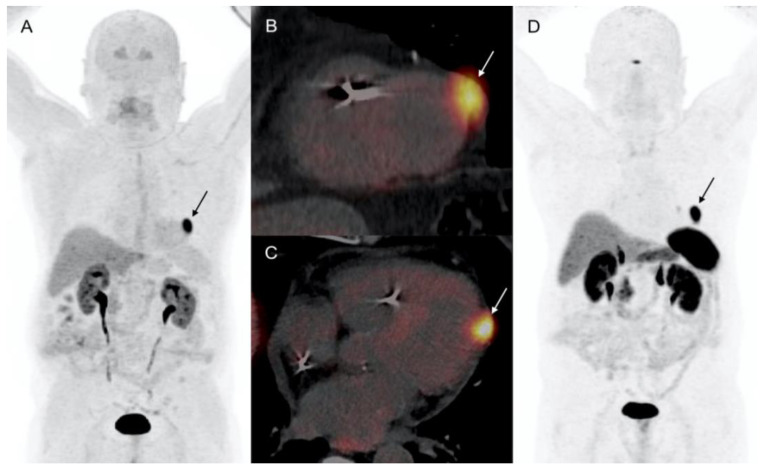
Patient 1. 72-year-old patient with metastatic si-NET. LV CM (arrows) showed intense uptake of both ^18^F-DOPA and ^68^Ga-DOTATOC. (A) ^18^F-DOPA PET MIP (anterior projection). (B,C) ^18^F-DOPA PET/CT short axis, and 4 chamber view. (D) ^68^Ga-DOTATOC PET MIP (anterior projection).
